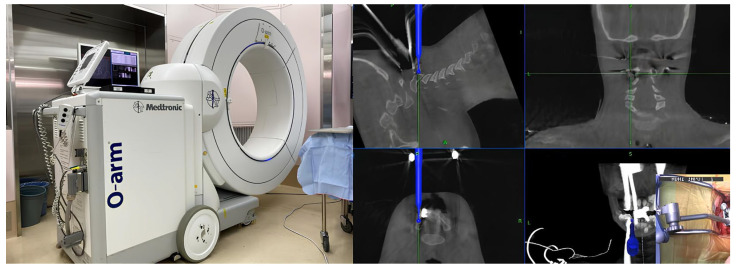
Intraoperative CT-based navigation.
